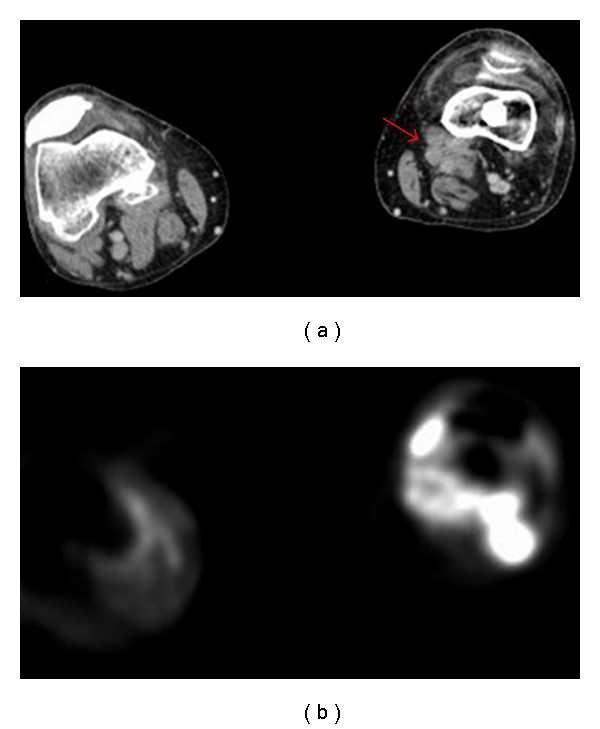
(a) A preoperative CT scan reveals multiple soft tissue nodules (arrow) above and below the knee including areas medial, lateral, anterior, posterior and in the suprapatellar recess. (b) A preoperative PET scan shows intense FDG uptake in the multiple soft tissue nodules above and below the knee consistent with recurrent disease.
